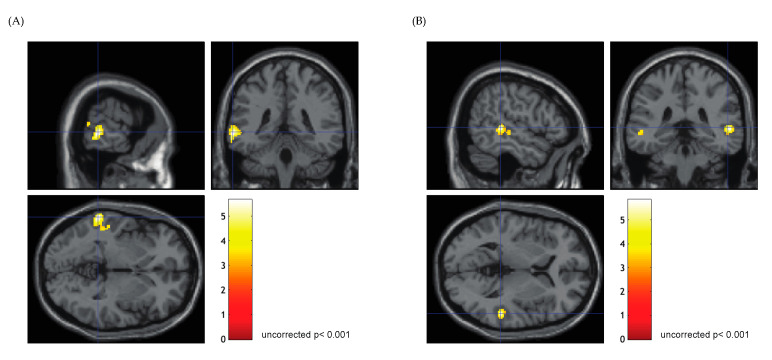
Functional magnetic resonance imaging activation in the time-reversed narrative condition. The crosshair line on the images indicates the coordinate of the local maxima. (A) In eighteen patients, passive listening to time-reversed narrative elicited significant activation in the left middle temporal gyrus or temporoparietal cortex. Significant activation distributed over the left posterior middle temporal gyrus was found in P4 (uncorrected *p* < 0.001). (B) Bilateral but right dominant activation was found in two patients (P8 is shown).
